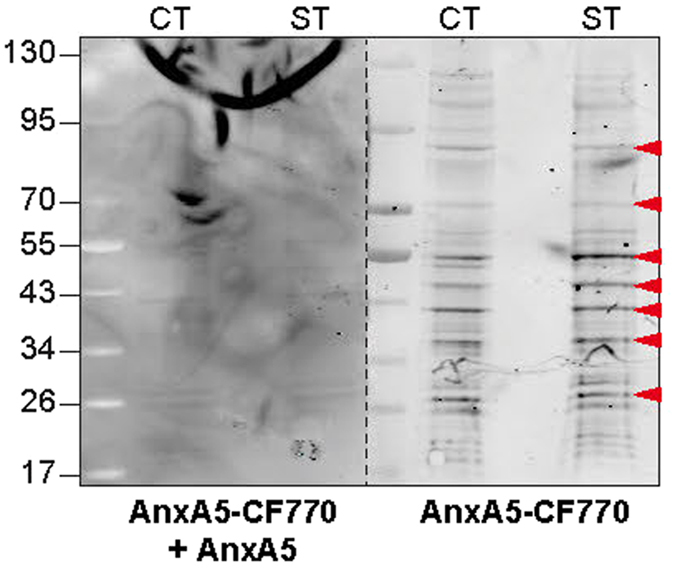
AnxA5 interactome at plasmalemma of human trophoblast. Proteins purified by sub-cellular membrane fractionation from cytrophoblasts (CT) or syncytiotrophoblasts (ST) were subjected to a solid phase binding assay-using AnxA5-CF770 (0.5 μM; AnxA5-overlay) as a probe in the absence or presence of an excess of unconjugated AnxA5 (1 μM). Red arrowheads indicate putative interacted-AnxA5 membrane proteins expressed in totrophoblasts.
